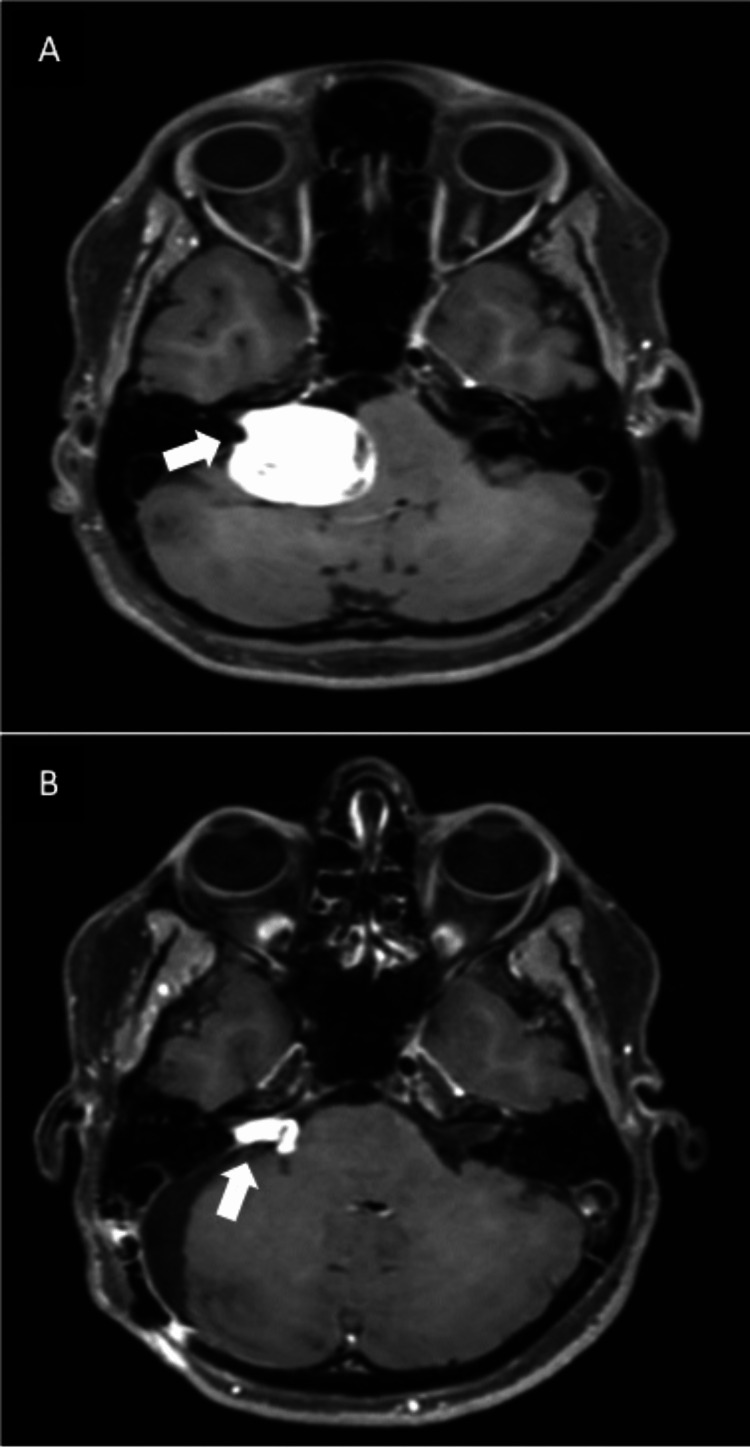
Pre-operative and post-operative MRI. (A) Preoperative axial MRI T1 gad imaging showing a voluminous extra-axial lesion centered on the right pontine-cerebellar angle cistern, extending from the plane of the trigeminal nerve/tent of the cerebellum to the plane of the jugular foramen, 33 x 28 x 29 mm anteroposterior, transverse and craniocaudal, respectively. The acoustic pore is enlarged. (B) Four months post-operative axial MRI in T1 Gad showed a right-sided tumoral residuum with intracanalicular and cistern components, 10 x 18 x 14 mm anteroposterior, transverse, and craniocaudal, respectively.
